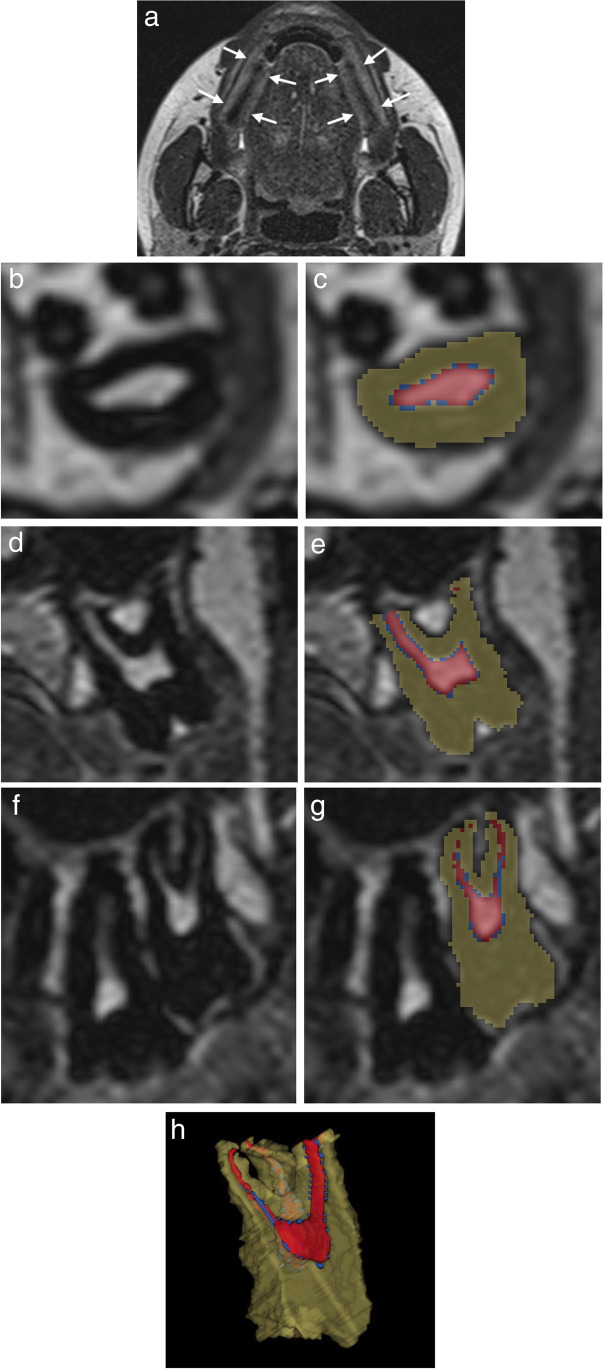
a Axial MRI shows correct bilateral placement of dental cotton rolls filled with water (arrows) between the molars. The cotton rolls delineated the upper and lower molars and stabilized the bite. b, c Axial MRI through the upper jaw shows unsegmented and segmented tooth 28. Green for hard tooth tissue, blue for predentine, and red for pulp. d, e Coronal MRI through the upper jaw shows unsegmented and segmented tooth 28. Green for hard tooth tissue, blue for predentine, and red for pulp. f, g Sagittal MRI through the upper jaw shows unsegmented and segmented tooth 28. Green for hard tooth tissue, blue for predentine, and red for pulp. h 3D rendering of the segmentation, with a wedge of approximately one quarter removed to visualize the pulp. Green for hard tooth tissue, blue for predentine, and red for pulp
